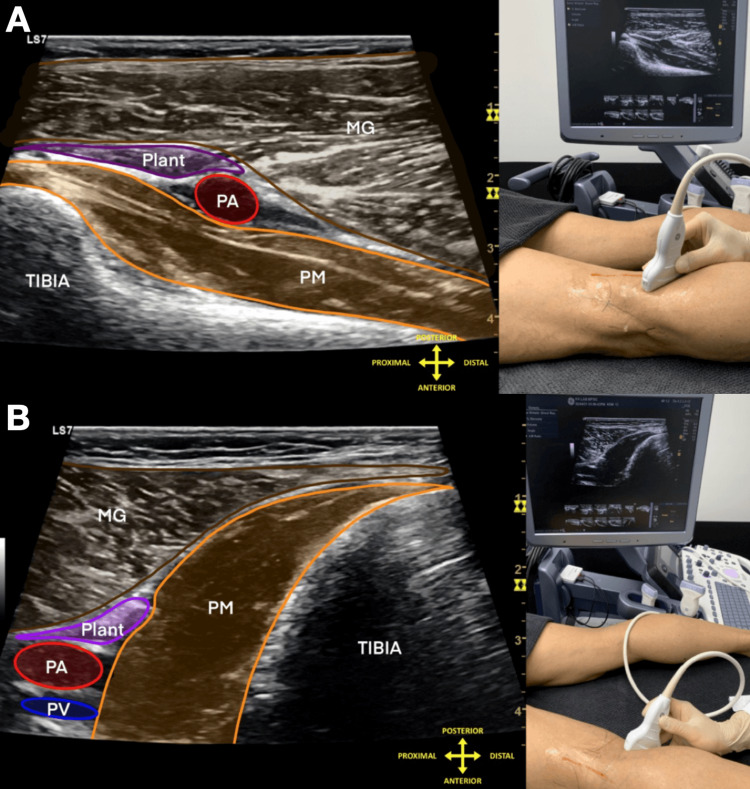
Oblique transverse long-axis ultrasound views of the popliteus muscle from the mid portion to its tibial insertion (A) Oblique transverse long-axis ultrasound image demonstrating the mid portion of the popliteus muscle.
